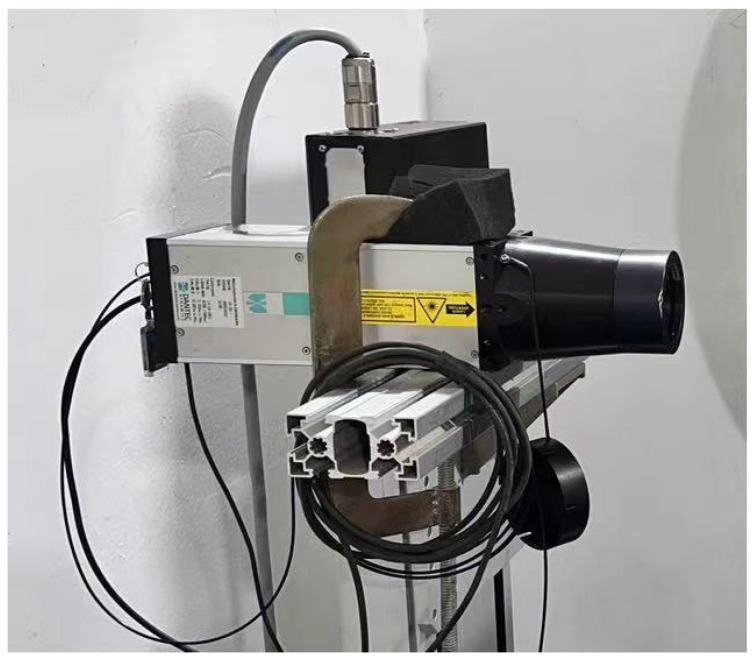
Laser Doppler Velocimetry.
